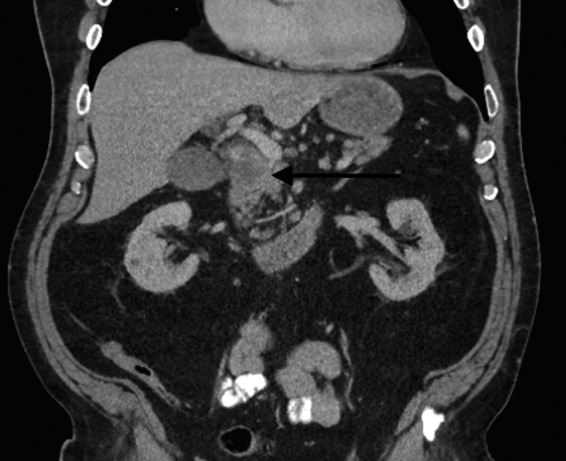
Coronal contrast-enhanced CT image demonstrates the hypo-attenuating mass in the head of the pancreas (*arrow*).
